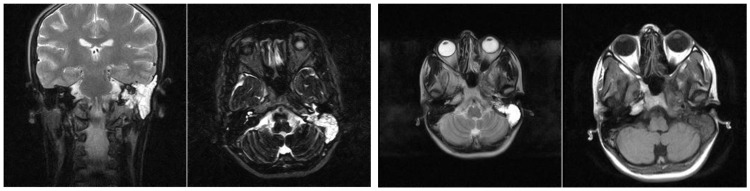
Patient with the diagnosis of chronic suppurative left retroauricular exteriorized chronic suppurative otomastoiditis, MRI examination with axial and coronal sections, with enlarged hyperseminal T2-weighted area in the middle ear and mastoid on the left side, hyposeminal T1-weighted.
